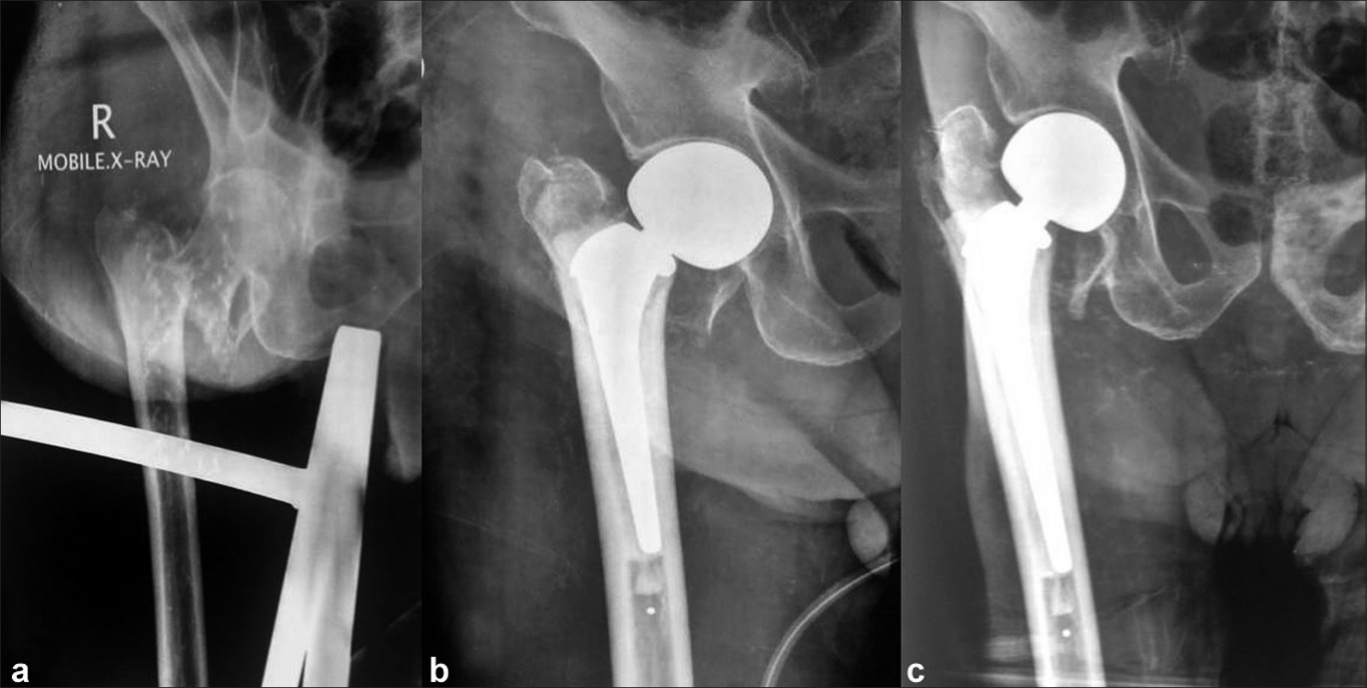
X-ray right hip joint anteroposterior view in (a) an 87 year old man with severe osteoporosis and unstable intertrochanteric fracture (b) treated with hemiarthroplasty (This patient had Alzheimer’s disease and refused to walk and eventually was wheelchair bound) (c) with adduction contrauture
